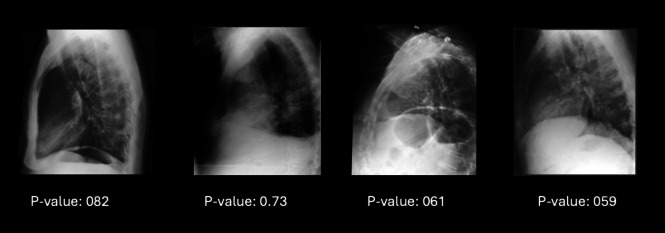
Example of lateral chest radiographs that failed in discrimination (as denoted by the *p*-values)
